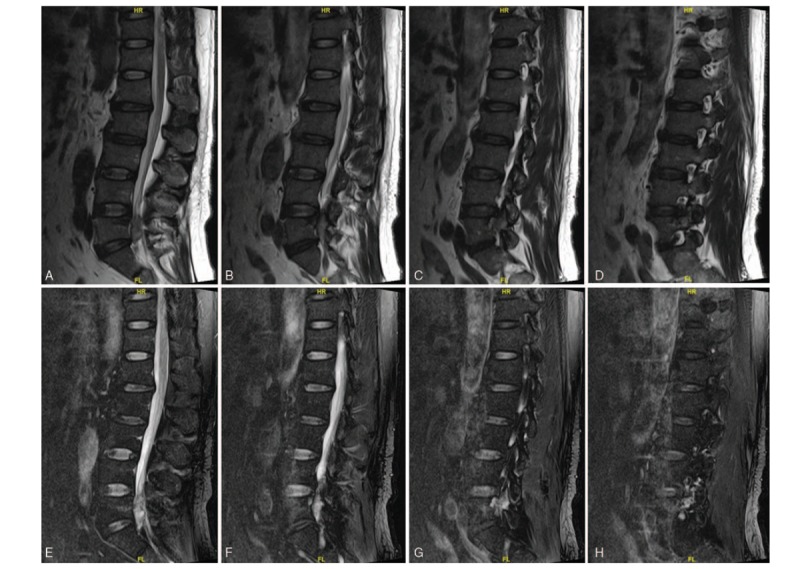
(A–H) Sagittal plane of the magnetic resonance imaging scan revealed the mass in the spine, which was highly indicative of the pathogenic tumor.
